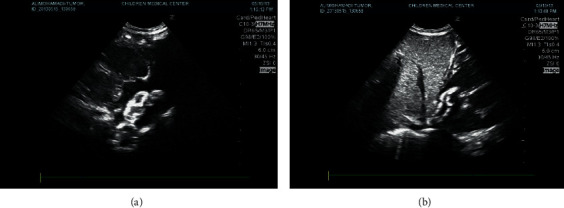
2D transthoracic, subcostal view demonstrating the echogenic mass (arrow head) attached to the interatrium septum (a) and extension to the IVC (b).
